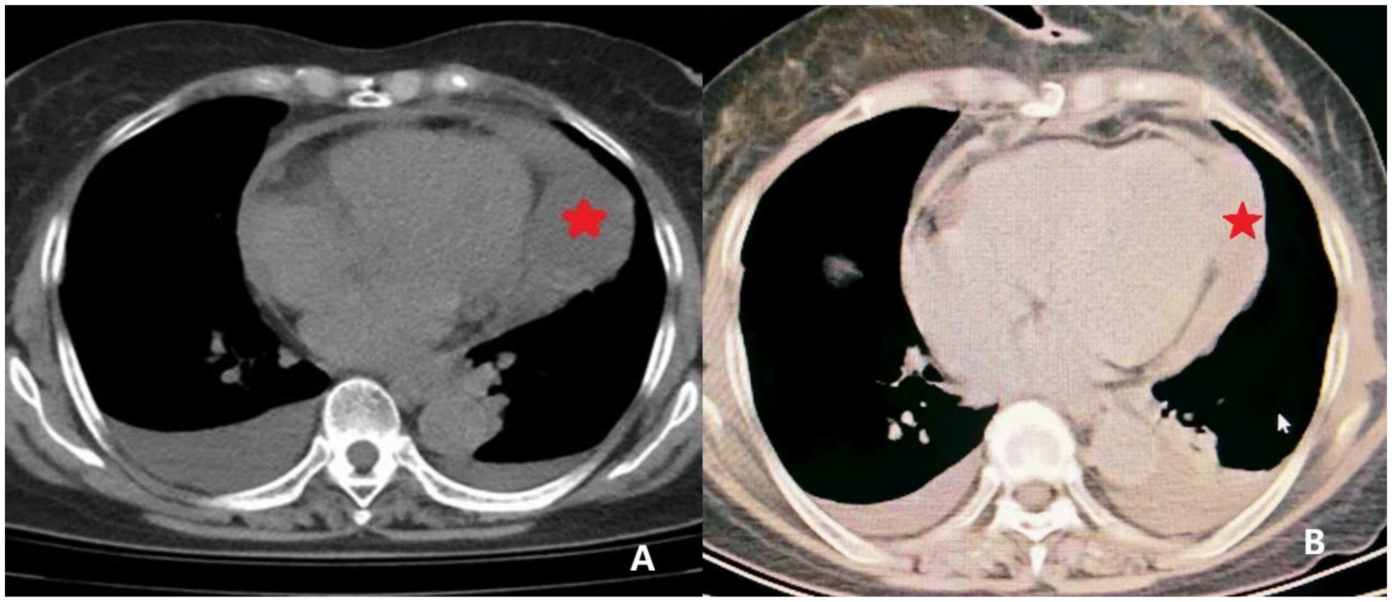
CT imaging of the pericardial mass. (A) Preoperative CT image revealing localized pericardial thickening with associated areas of fluid density. A focal soft tissue density is noted at the cardiac apex (indicated by *) concerning for a space-occupying lesion. (B) Postoperative CT image demonstrating resolution of the pericardial thickening and absence of the previously noted soft tissue density at the cardiac apex, indicating successful resection.
